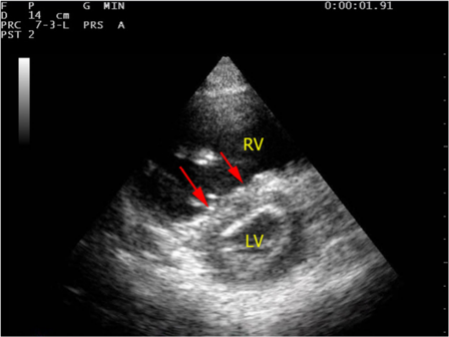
Acute cor pulmonale due to massive pulmonary embolism. A parasternal short axis view shows a dilated RV and D-shaped LV on parasternal view in a patient with massive pulmonary embolism. The flattened IVS is highlighted by *red arrows*. (*RV* right ventricle; *LV* left ventricle)
